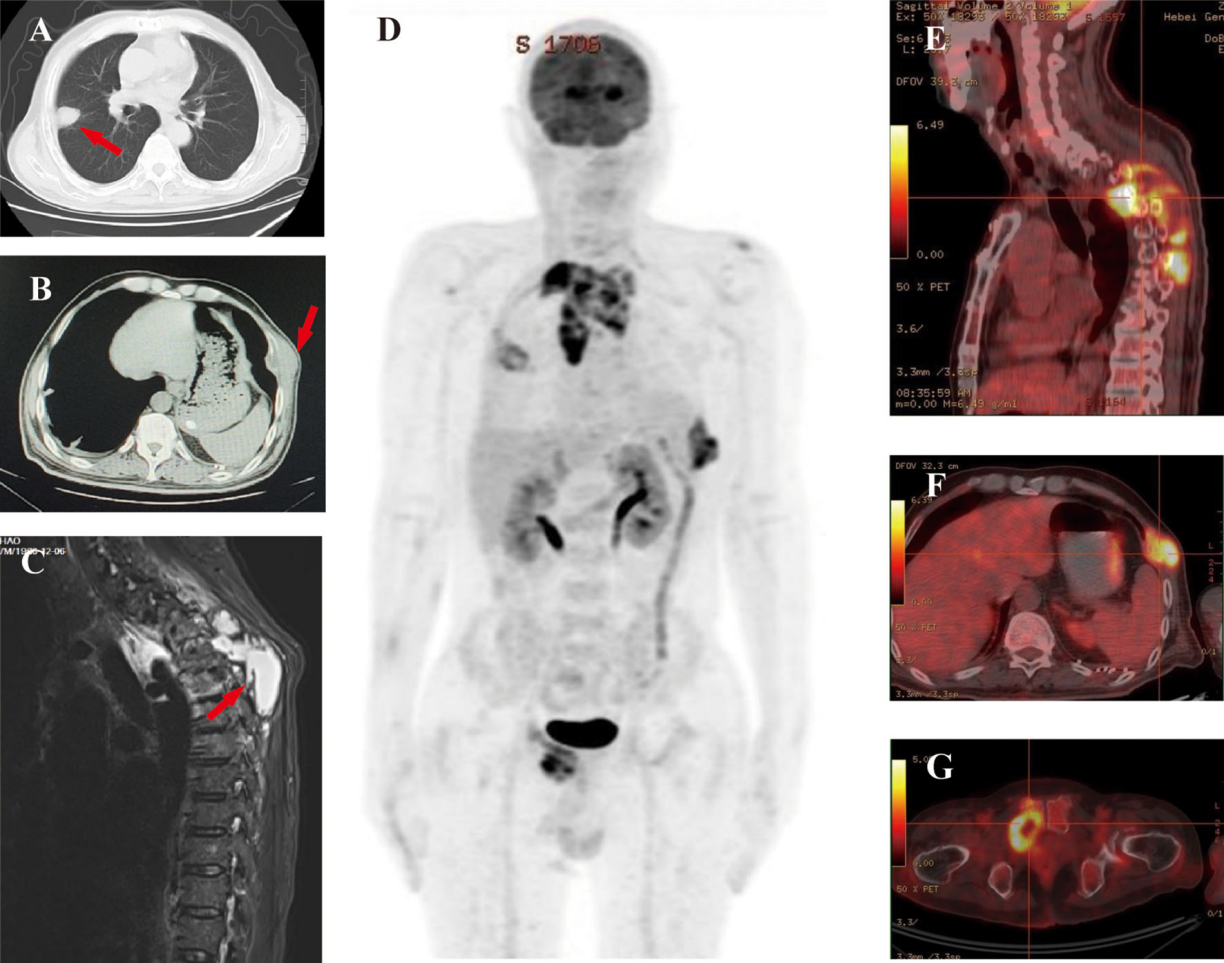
Radiological imaging of Case 1. (A) Chest CT demonstrates a 1.2 cm nodule (arrow) in the right upper lobe with surrounding ground-glass opacity. (B) Chest CT reveals localized left pleural thickening and an 8 cm chest wall mass (arrow). (C) Sagittal T2-weighted MRI of the thoracic spine shows inflammatory changes involving T3-T5 vertebral bodies and surrounding tissues with pathological compression fracture at T4 (arrow). (D) PET-CT further identified widespread hypermetabolic lesions, with SUVmax of 10.6 in the upper thoracic region (E), 8.2 in bilateral chest walls (F), and 9.5 in the right pubic ramus (G).
